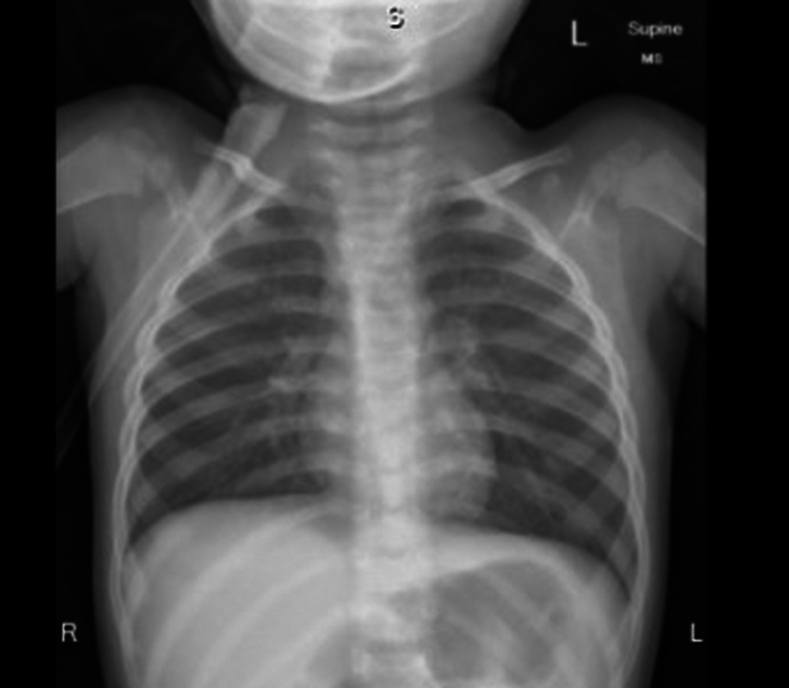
X-ray chest shows mild hyperinflation of left lung.
